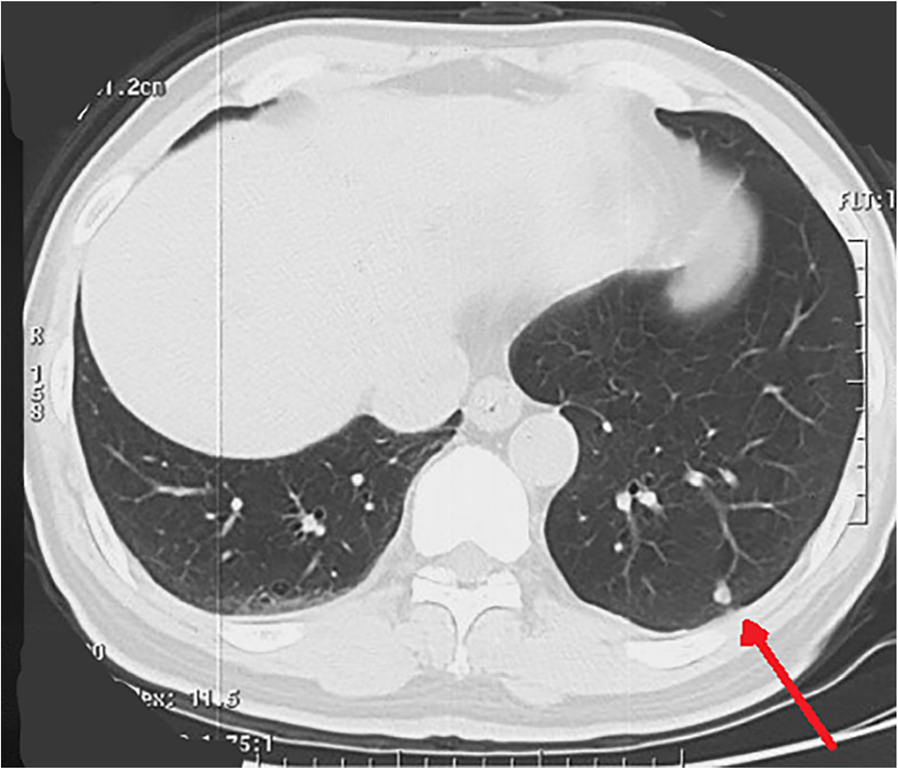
Axial computed tomography image reveals a nodule (0.7 × 0.6 cm) in the left lower lobe (arrowhead)
